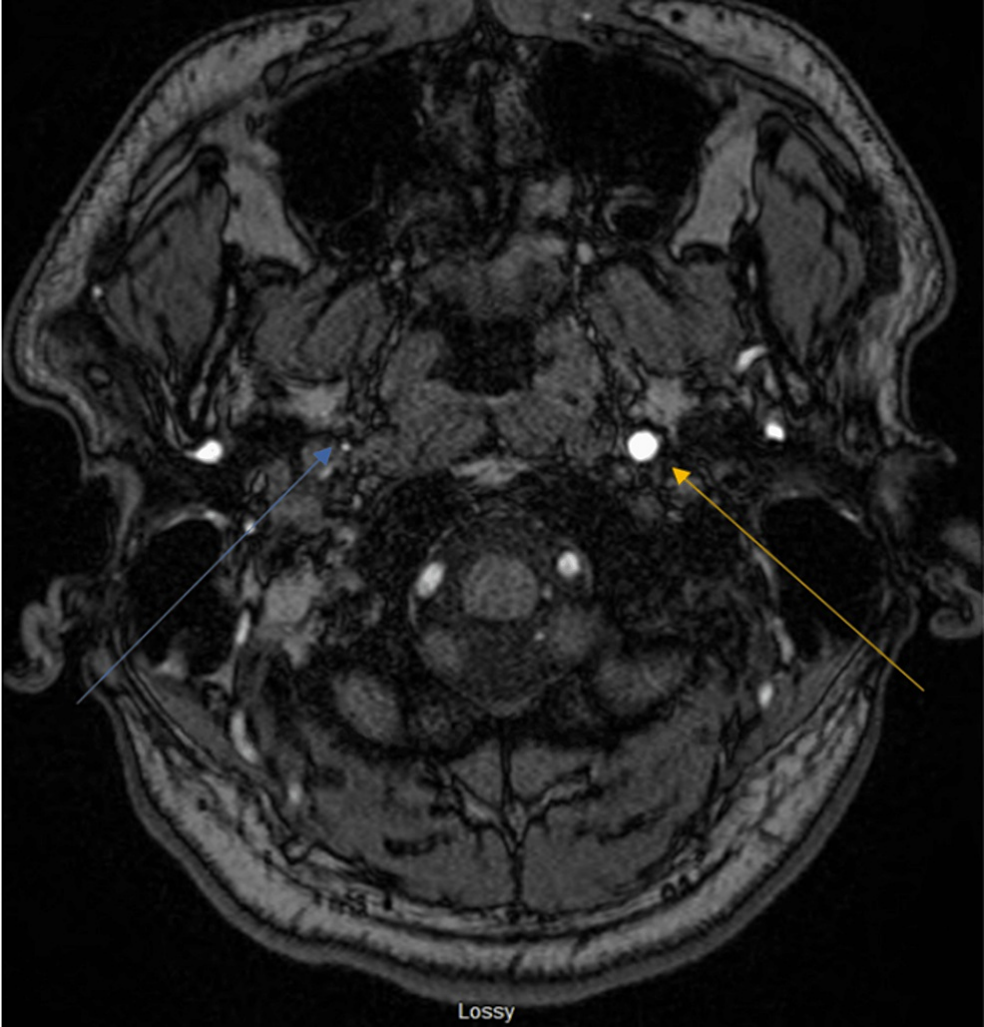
Magnetic resonance imaging of the brain. The blue arrow indicates dissection without flap and clotted blood completely filling the false lumen at the skull base versus intramural hematoma with critical narrowing at the skull base. The yellow arrow reveals normal anatomy.
